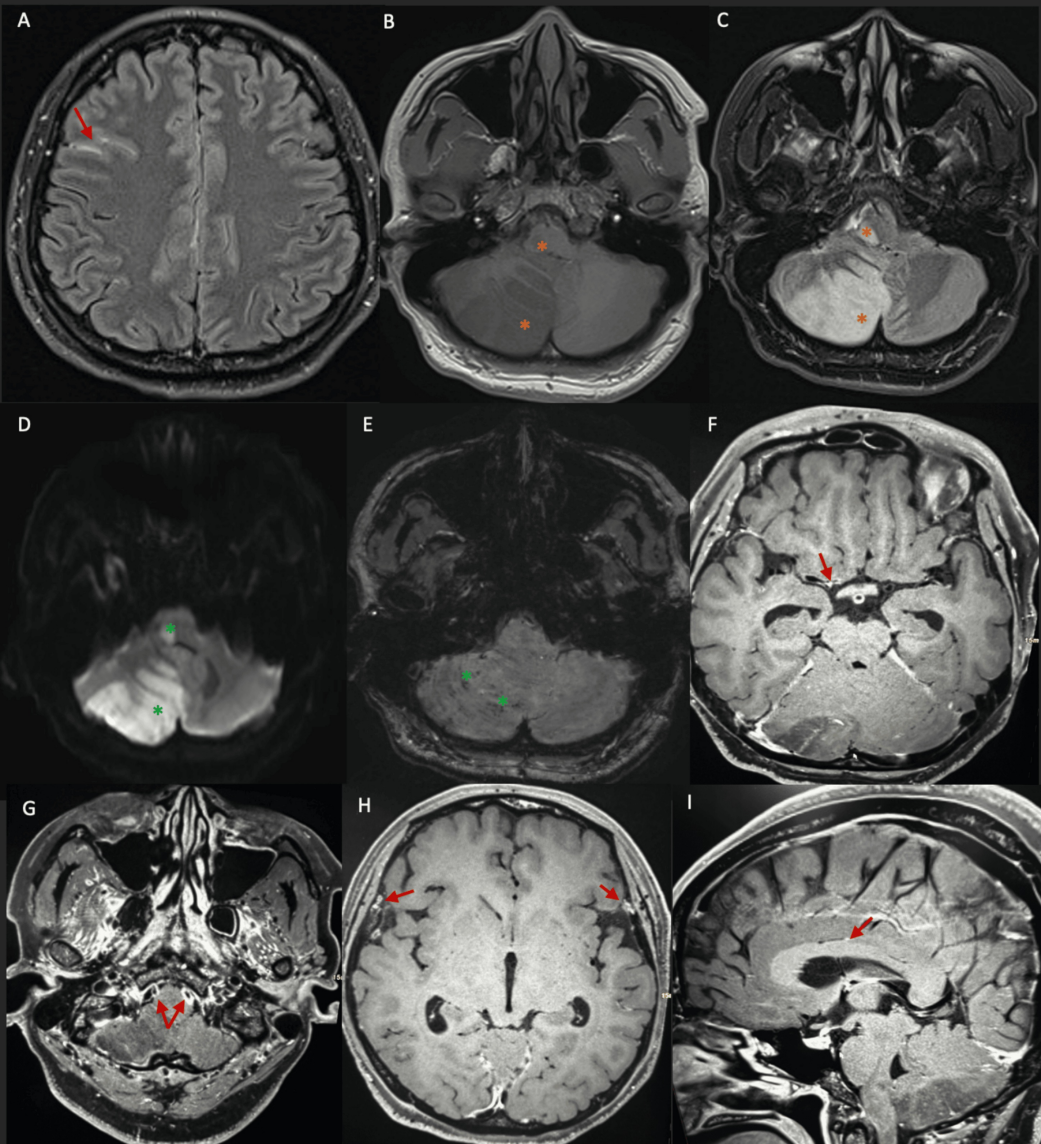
An MRI of the brain with contrast and vessel wall imaging (A-F) (A) Fluid-attenuated inversion recovery (FLAIR) with contrast images demonstrating minimal right frontal subarachnoid hemorrhage (red arrow). (B-E) Large areas of low T1 (B) and high T2/FLAIR (C) signal changes involving the right cerebellar hemisphere and right lateral medulla (orange asterisks). The corresponding areas demonstrate diffusion restriction on diffusion-weighted imaging (DWI) (D) and minimal blooming on susceptibility-weighted imaging (SWI) sequences (E) (green asterisks), suggesting acute infarcts with microhemorrhages. (F-I) High-resolution vessel wall imaging demonstrates smooth, moderate luminal narrowing with concentric wall thickening and enhancement involving multiple intracranial arteries, including the bilateral vertebral arteries, the supraclinoid segment of the right internal carotid artery, the right M1 segment, bilateral middle cerebral artery cortical branches, and the pericallosal branches of the right anterior cerebral artery (red arrows), findings consistent with inflammatory small- and medium-vessel vasculitis.
